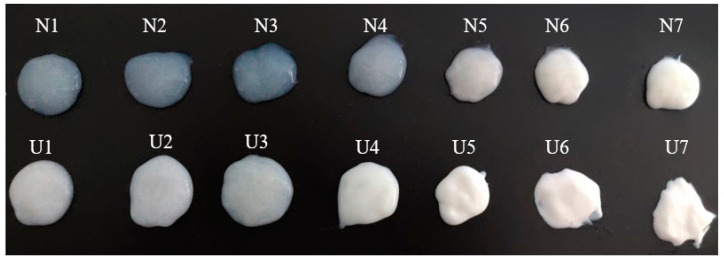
The profiles of emulsions (30 g·L^−1^) with non-ultrasound and ultrasound emulsification treatment.
